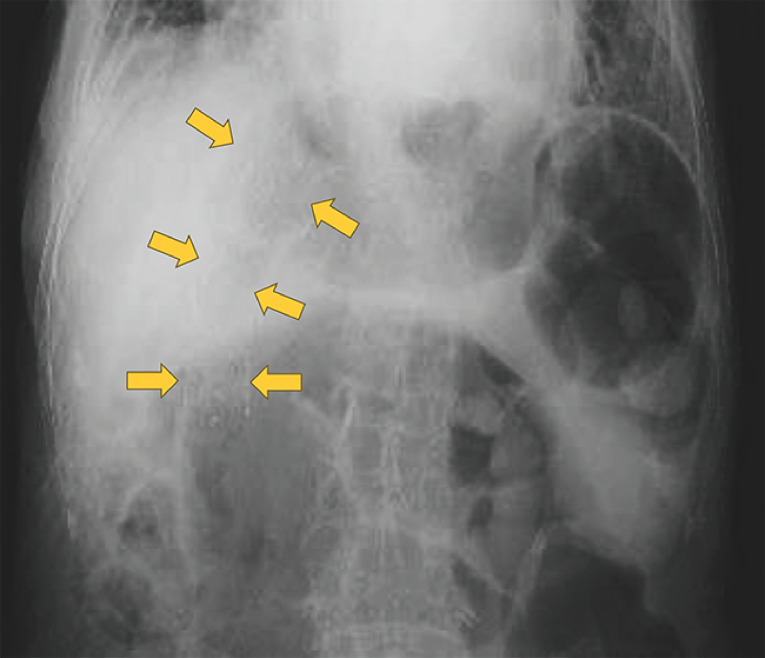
Radiographic image taken 2 weeks later showing no evidence of stent migration.
